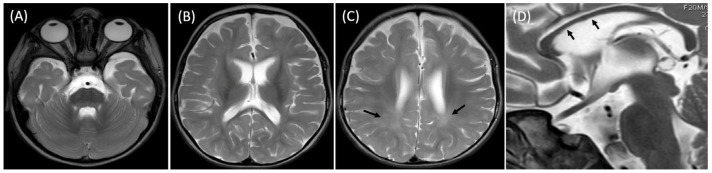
Axial T2-weighted brain MR images (A–C) showing anterior temporal hypoplasia (A) and widened pericerebral fluid spaces (B,C) indicating cortical volume loss as well as mild ventriculomegaly (B,C) consistent with white matter atrophy and periventricular T2-hyperintensities (C, arrows) suggestive of delayed white matter myelination. Sagittal T2 weighted image (D) showing thin corpus callosum (arrows).
